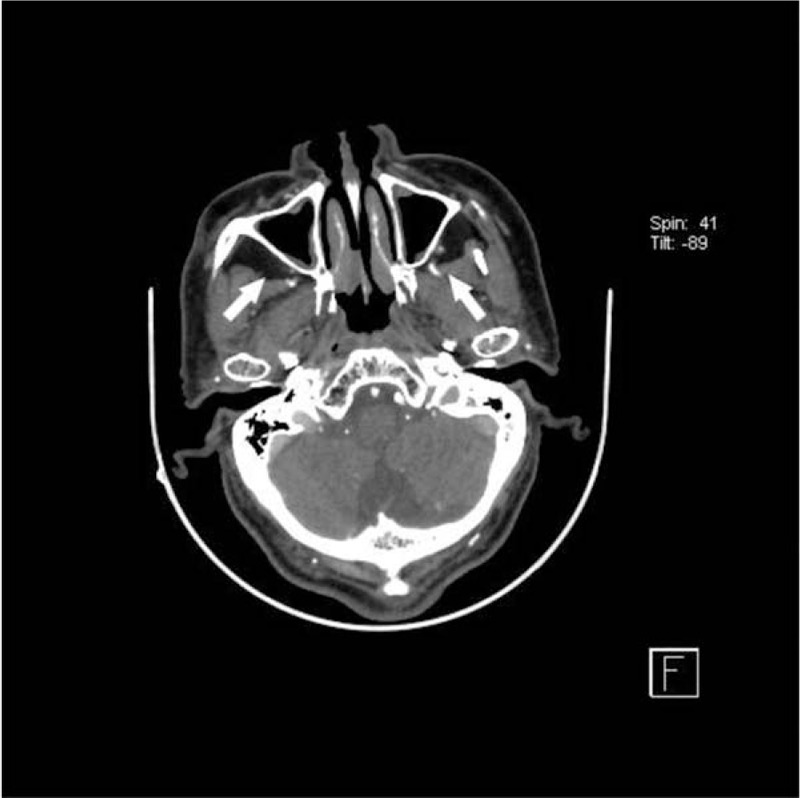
Axial (A) CTA image demonstrating inflammation in both maxillary sinuses (white arrow). This incidental finding was initially reported on neck CTA.
